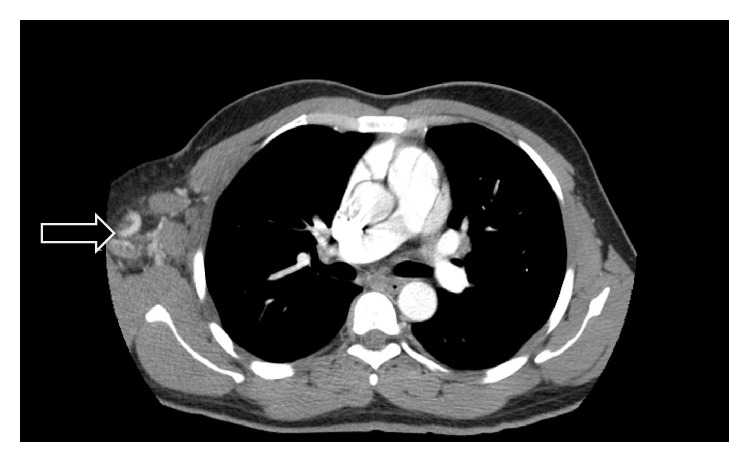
Computed tomography showing extensive right axillary lymphadenopathy (arrow).
